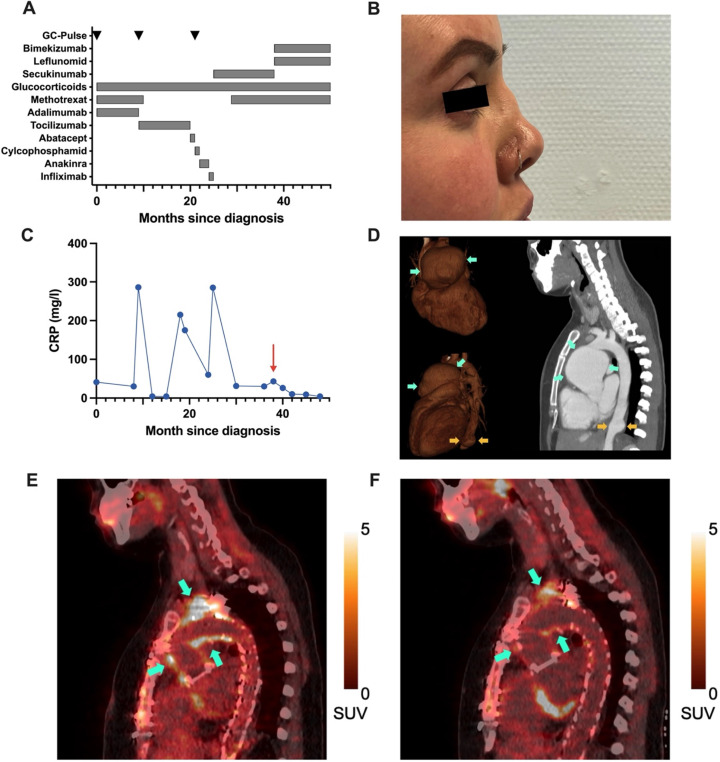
(A) Sequential immunotherapy during the clinical course. (B) ‘Saddle-nose’ deformity due to severe destruction of nasal cartilage. (C) C-reactive protein (CRP) level during the clinical course, the arrow indicates time at bimekizumab initiation. (D) Three-dimensional reconstruction of the heart and large intrathoracic vessels in frontal (upper left) and left lateral (lower left) view based on contrast-enhanced computed tomography of the thorax (portal venous phase). ‘Maximum intensity projection’ of the same image (right) in parasagittal slice guidance (5-mm slice thickness, 1-mm slice distance). Image of an aneurysm of the ascending thoracic aorta measuring max. of 8.5 cm (blue arrows) and an aneurysm of the descending thoracic aorta measuring max. of 2.7 cm (yellow arrows). E, F, 18F-fluorodeoxyglucose ([18F]FDG) positon emission tomography-computed tomograühy PET/CT images showing FDG-uptake before (at month 33, during secukinumab therapy) (E) and after (at month 48) (F) treatment with bimekizumab, with a significant decrease in metabolic activity in the thoracic aorta. SUV = standardized uptake value.
